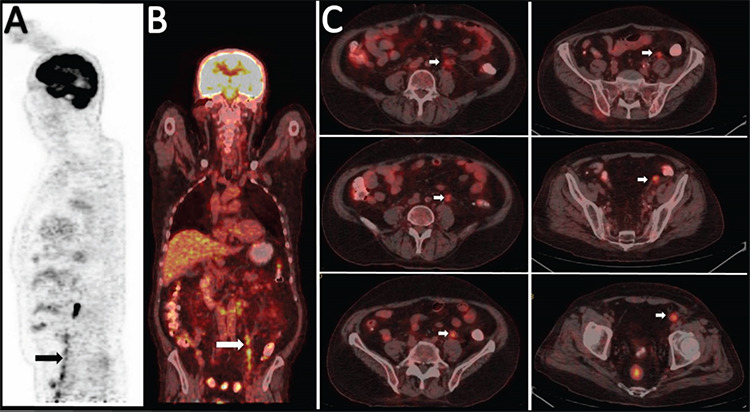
(A-C) F18-FDG PET/CT after left orchiectomy depicted moderate hypermetabolic metastatic retrocrural and paraaortic lymph nodes and multiple intense hypermetabolic foci along the course of the left testicular vein extending up to the left renal vein suggesting testicular vein infiltration of myeloid sarcoma (arrows). F18-FDG PET/CT: F18-fluorodeoxyglucose positron emission tomography/computed tomography.
